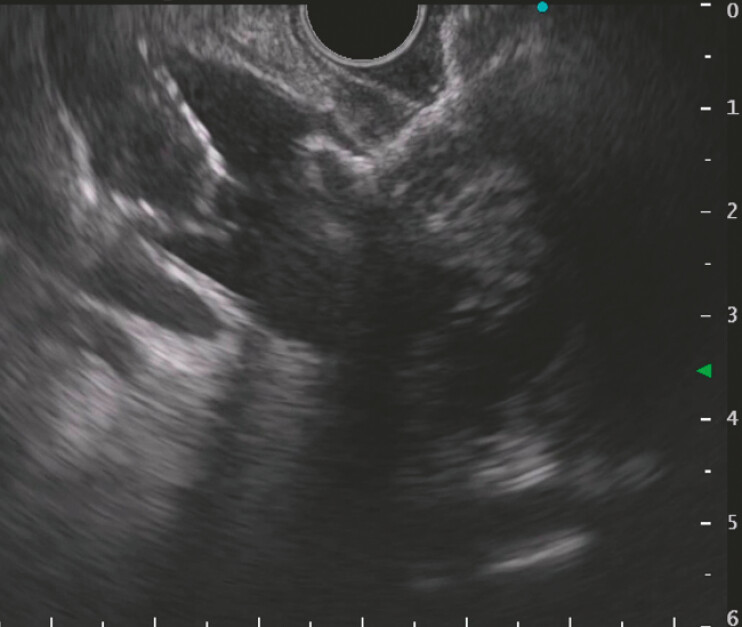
EUS-guided deployment of a 6 × 8 mm Hot LAMS for choledochoduodenostomy. EUS, endoscopic ultrasound; LAMS, lumen-apposing metal stent.
